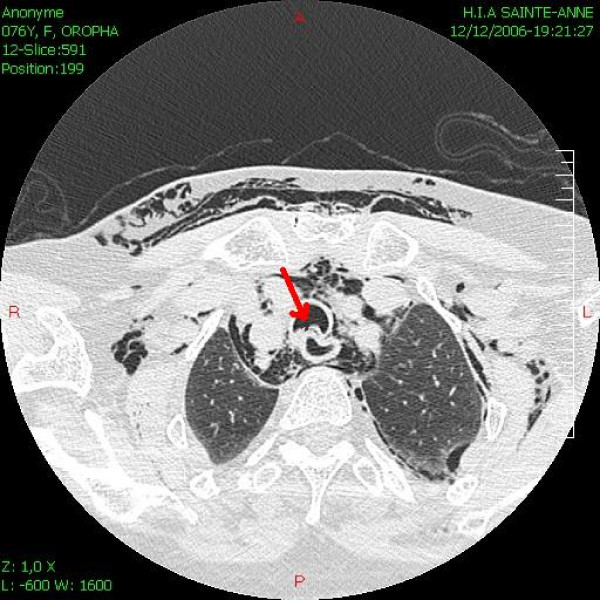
Thoracic CT showing posterior tracheal rupture (Arrow).
